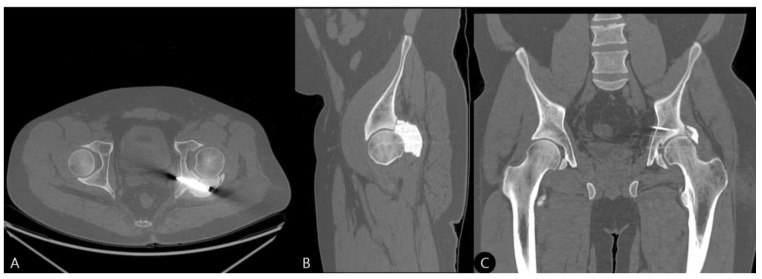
Fifteen-month postoperative follow-up CT scans. (A) Axial, (B) sagittal, and (C) coronal views showing a stable hip joint without signs of subluxation or loosening.
